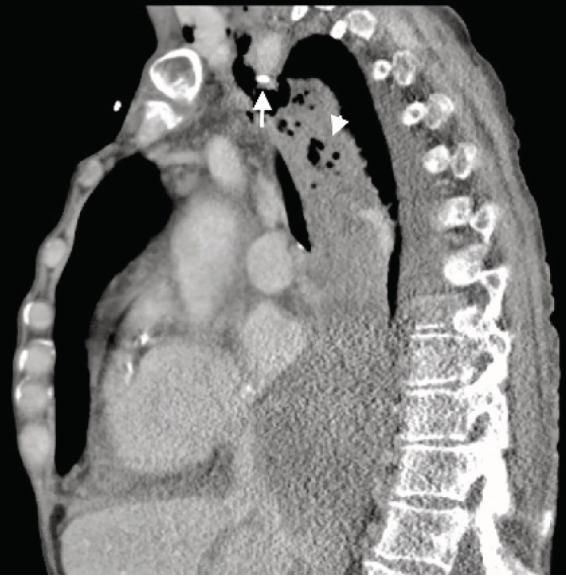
Sagittal computed tomography of the thorax demonstrating gas and fluid collections in posterior mediastinum (arrowhead) with peripherally inserted central catheter tip (arrow) terminating extraluminal in superior mediastinum.
